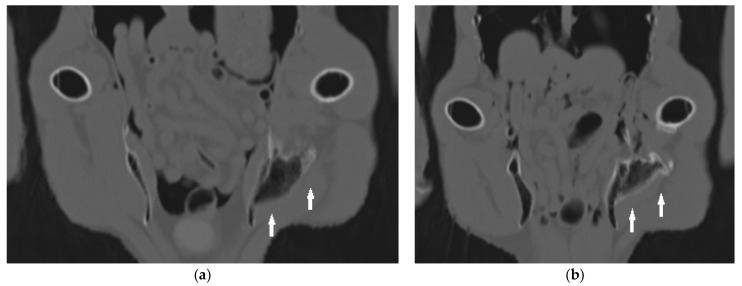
Horizontal computed tomographic images of a bald eagle (case 2) at the level of the pelvis and femur: more ventral (a) and more dorsal (b). Heterotopic ossifications in the course of the adductors (arrows) show fusion with the pelvis (a,b) and the femur (b). The large bone formation attached to the pelvis resembles a mature lamellar bone. In addition, the irregular structure of the musculature can be seen adjacent to the bone formations, especially in comparison to the healthy side.
